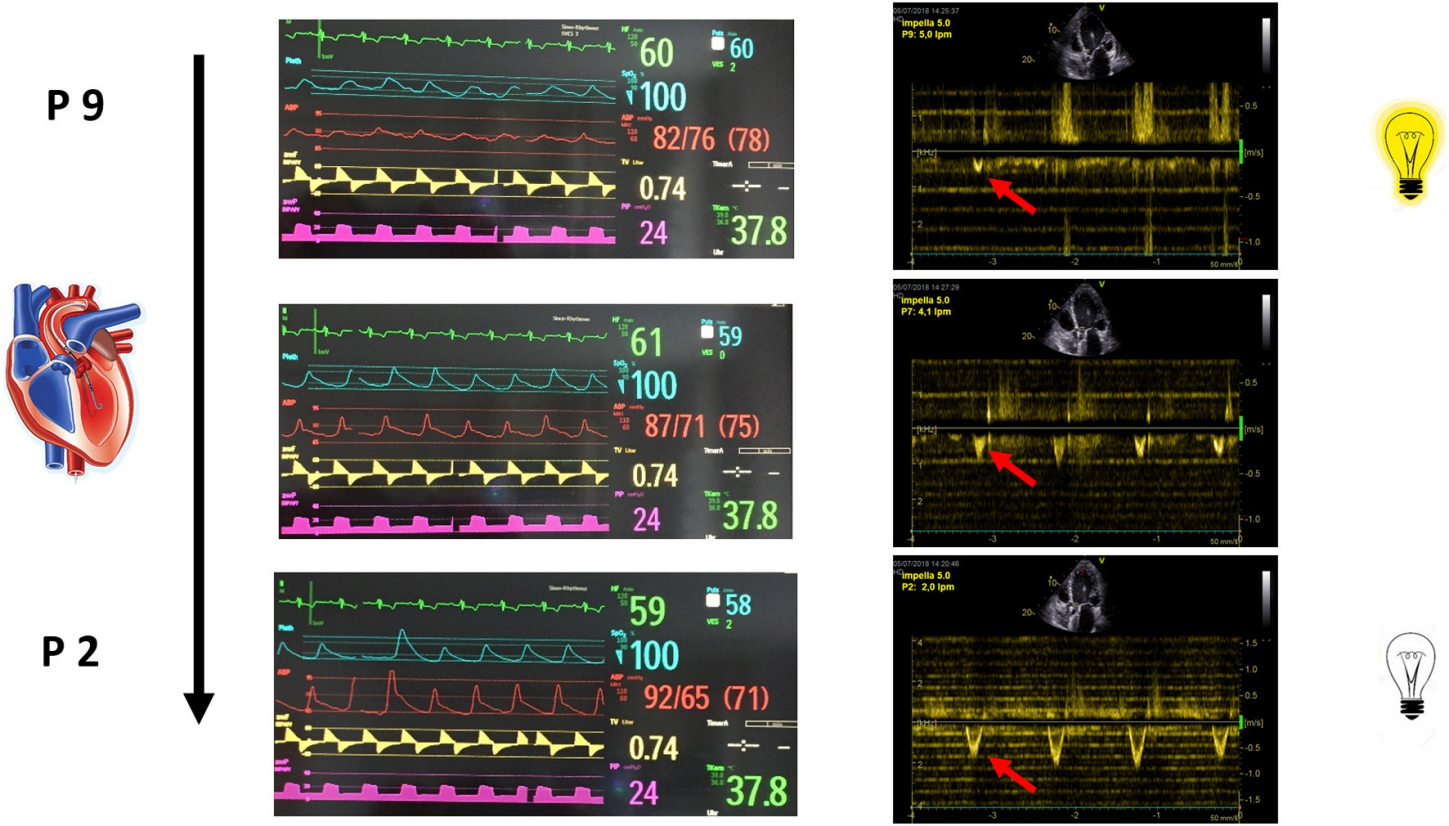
The increase in LVOT VTI with a lower level of Impella support is the Doppler echocardiographic demonstration of bi-ventricular recovery.
